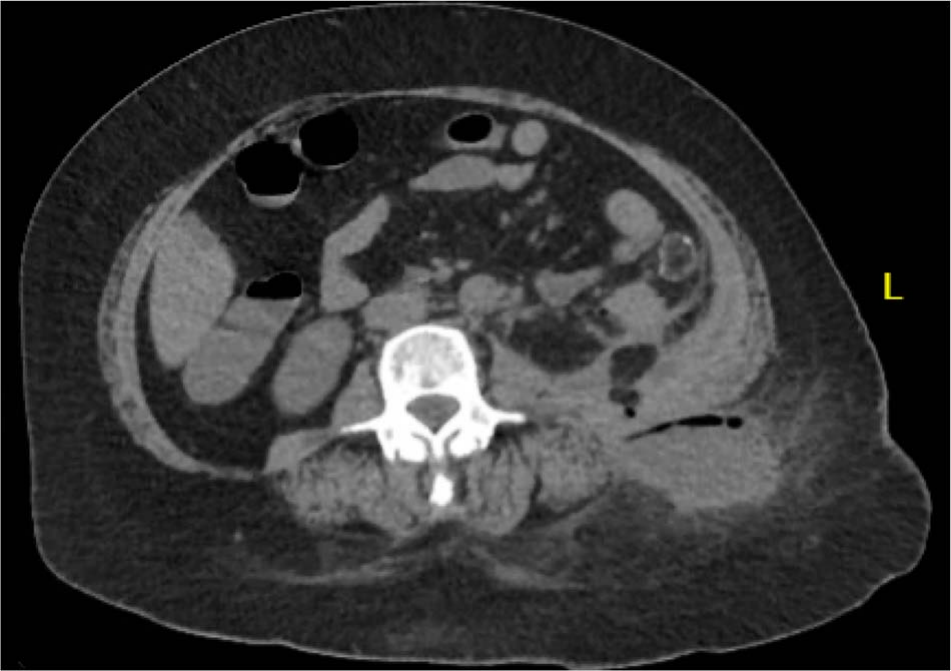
Patient A. Initial CT abdomen and pelvis showing left subcutaneous fluid collection extending intra-abdominally.
